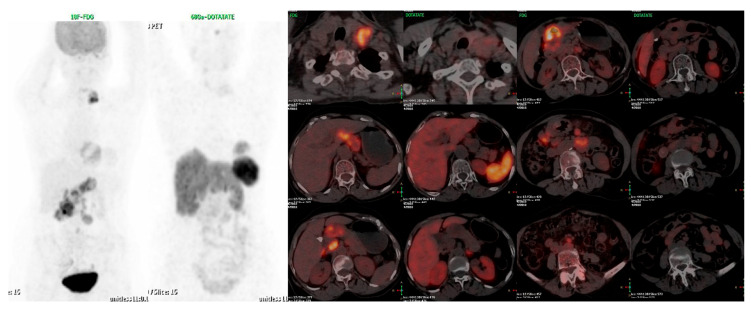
A 63-years-old female patient, recently diagnosed with Gastro-Duodenal NET, presented with pain abdomen, iron deficiency anemia and unexplained weight loss (4 kg). Gastric biopsy was suggestive of poorly differentiated NEC, WHO grade III, IHC: Synaptophysin positive; Chromogranin-A and CD-56 negative; Ki-67 index 80%. Dual tracer PET-CT with FDG and DOTATATE showed no increased DOTATATE uptake (Krenning score 0 to 1) noted in the pyloric antral mass with severe luminal narrowing and proximal dilatation with multiple enlarged lesser sac and peripancreatic lymph nodes. metabolically active gastro-duodenal primary and left supraclavicular, abdominal and retroperitoneal nodes, suggestive of minimal to no SSTR expression in the lesions. This is consistent with poor differentiation of the tumour/high Ki67 index of 80%. Hence, dual tracer PET-CT scan has potential to ascertain tumour biology and to guide best personalized management for the patient with NENs.
